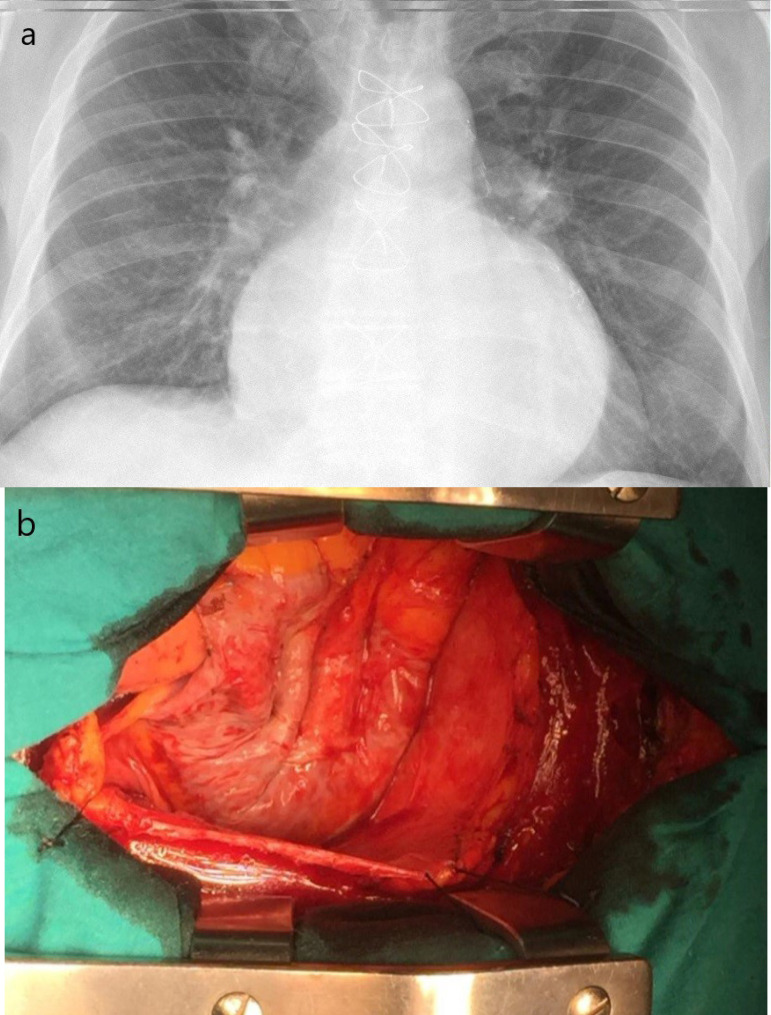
Decompression of huge right atrium with peripheral cardiopulmonary bypass. A) Preoperative chest X-ray; B) decompressed right atrium.
